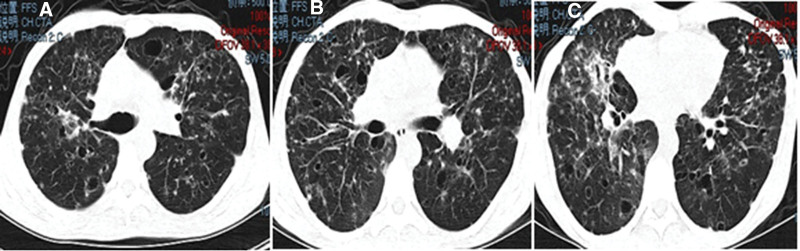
Repeated chest computed tomography on March 1, 2021 showing multiple cysts, <1 cm in diameter, and nodules distributed in both lungs. (A) Both upper lung levels; (B) tracheal bifurcation level; (C) both lower lung levels.
